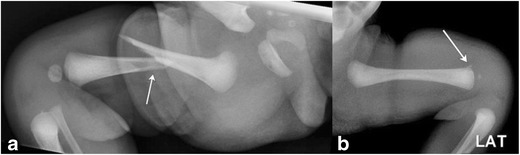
Femur fractures Frontal radiograph of the lower extremity in a 1-day-old infant (a) demonstrates displaced an oblique mid-shaft fracture of the right femur. This was a consequence of excessive traction on the femur. Lateral radiograph of the femur (b) in another 1-day-old breech infant demonstrates irregularity at the distal femoral metaphysis, which was proved to be a birth-trauma related physeal injury with chondroepiphyseal separation at the distal femur on the subsequently performed MR (images not included)
